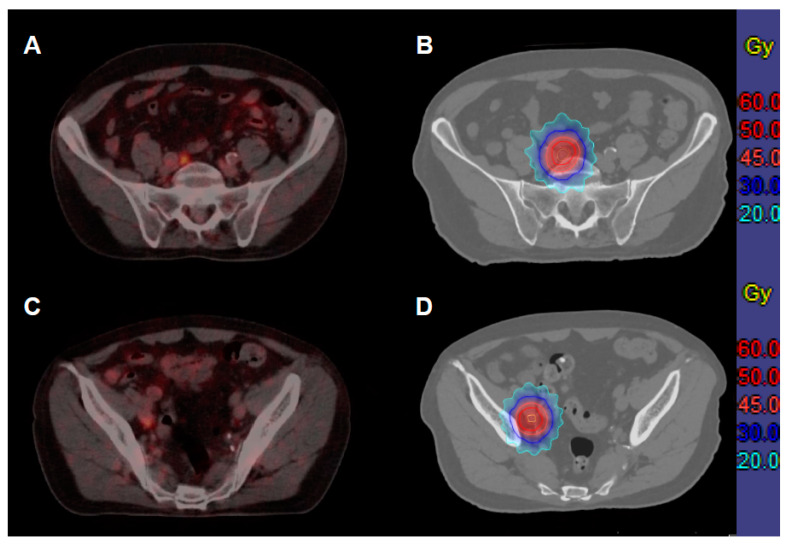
A 75-year-old patient with intermediate-risk prostate cancer (PSA 13.35 ng/mL, Gleason’s score 4 + 3 = 7) received primary radiation therapy (76 Gy in 2 Gy fractions). After three years, he developed a biochemical failure (3.4 ng/mL), and the PSMA-PET-CT showed two iliac lymph node metastases (A,C), which were treated with ablative radiotherapy (50 Gy in 2 Gy fractions, (B,D) gross tumor volume depicted in yellow, clinical target volume in orange, planning target volume in red. Until five years after treatment (end of study), no recurrent disease was detected.
